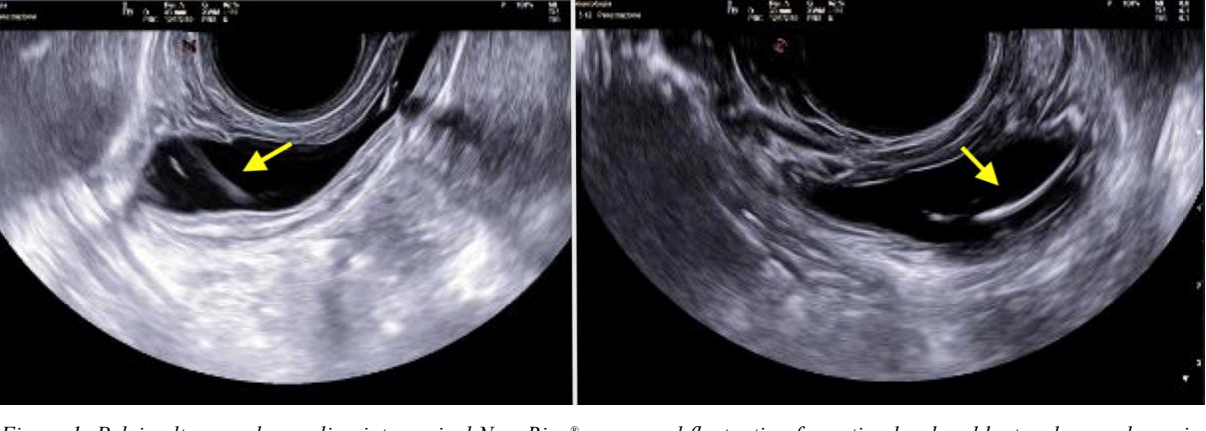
Pelvic ultrasound revealing intravesical NuvaRing® as an oval fluctuating formation bordered by two hyperechogenic, non-vascularized lines.
